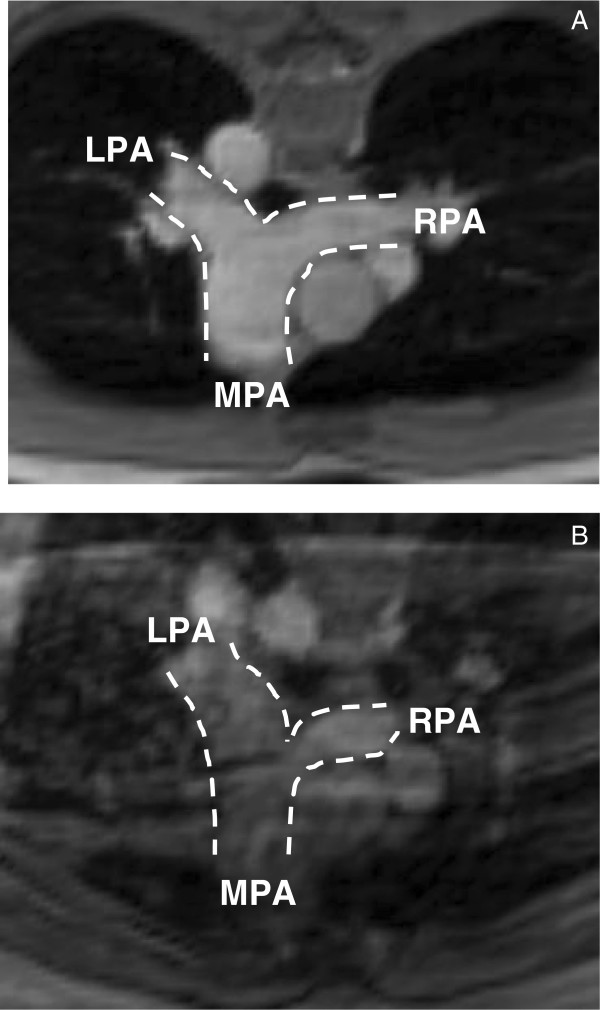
The detail of PA anatomy confirmed by the magnitude image of 4D PC MRI for the subjects, A) control and B) patient.
